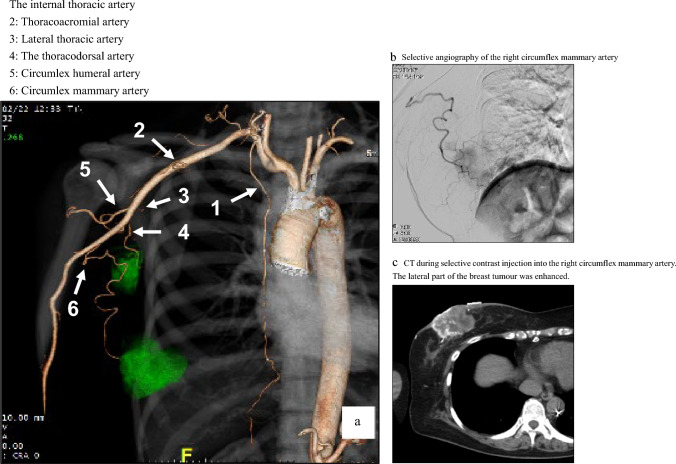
Right breast angiography and selective arterial CT. Arrows The internal thoracic artery 2: Thoracoacromial artery 3: Lateral thoracic artery 4: The thoracodorsal artery 5: Circumlex humeral artery 6: Circumlex mammary artery a. Angio-image of the right subclavian artery via the volume rendering technique, b. Selective angiography of the right circumflex mammary artery. c. CT during selective contrast injection into the right circumflex mammary artery. The lateral part of the breast tumour was enhanced
